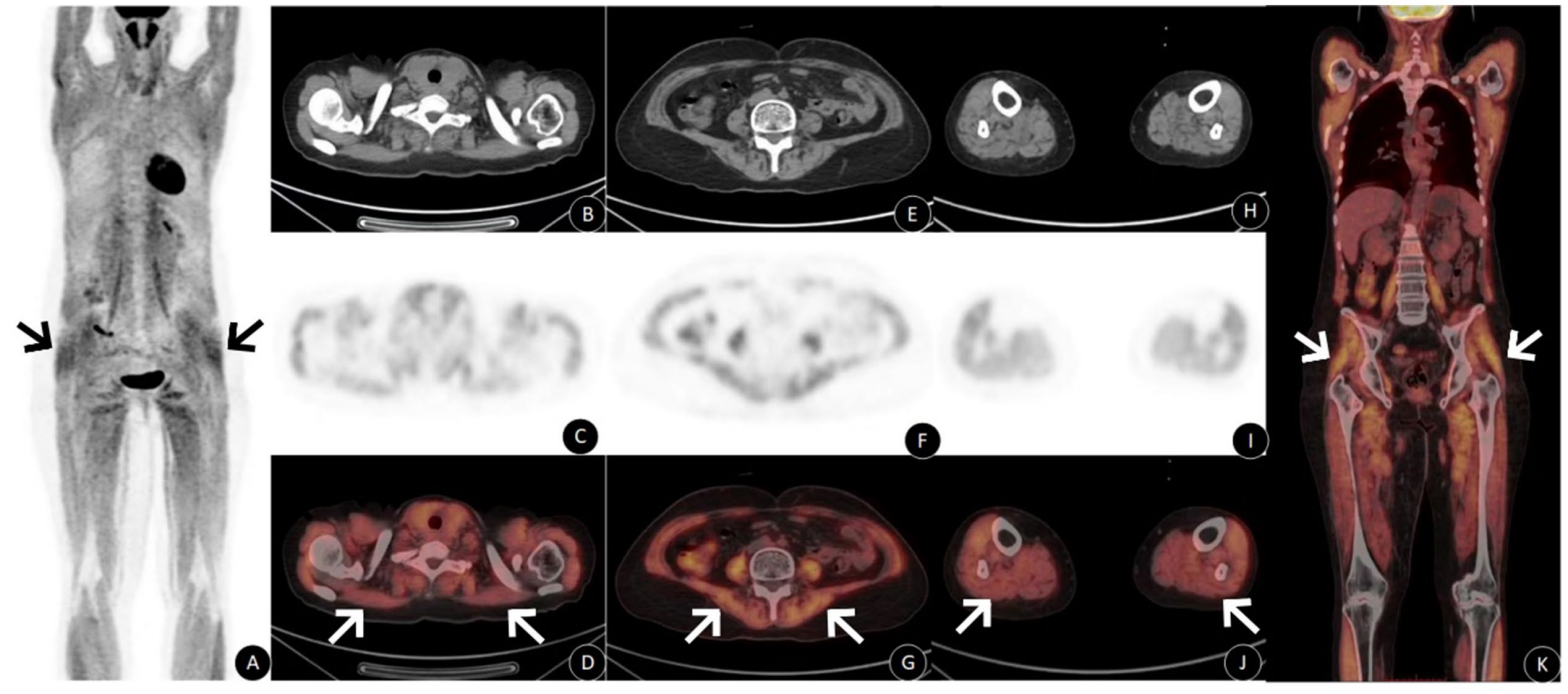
Panel (A) shows diffuse increased [^18^F]FDG metabolism in whole body muscles; panel (B–J) shows increased muscle metabolism in shoulder, lower back, and lower limbs in PET and PET/CT fusion images, but no obvious abnormality was found in CT images. Panel (K) shows the coronal fusion map, and the diffuse metabolism of the whole body muscles is increased.
